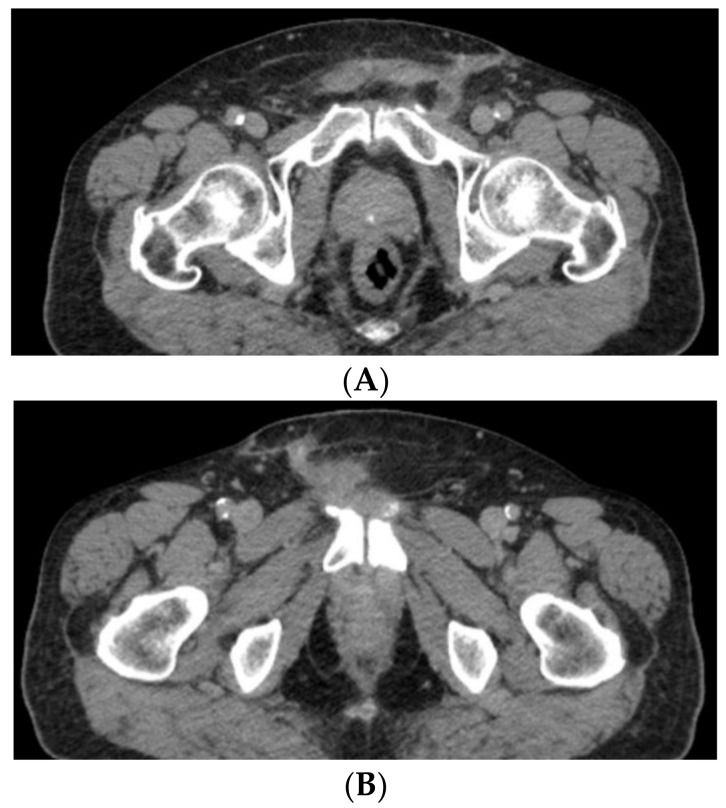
CT scan aspect, over three months after the tumor resection, at various levels, axial sections (A,B).
